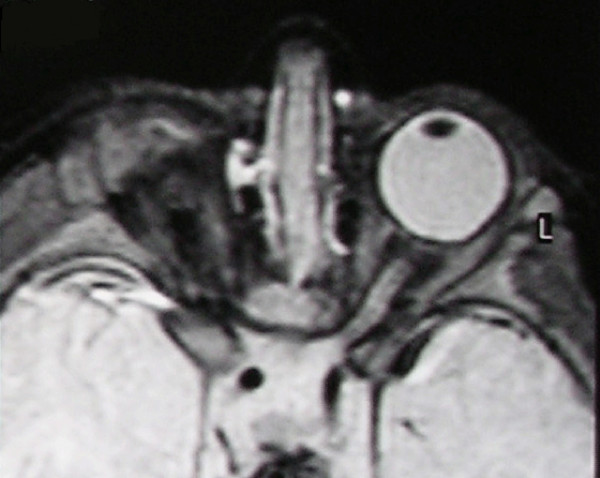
T2-weighted MR scan of a patient with unilateral anophthalmia. Note the presence of amorphous tissue and structures resembling extraocular muscles within the anophthalmic right orbit. The right optic nerve/chiasm junction appears attenuated rather than absent suggesting possible residual optic nerve neural tissue.
